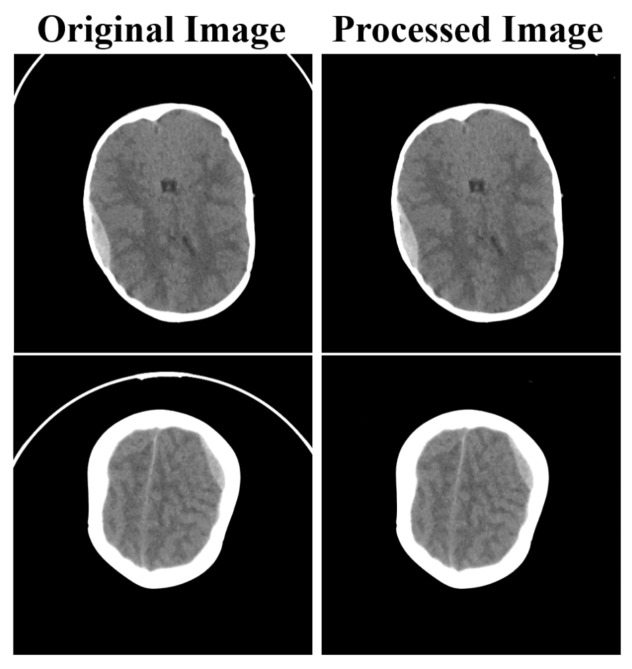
Removal of artifact regions in CT images.
